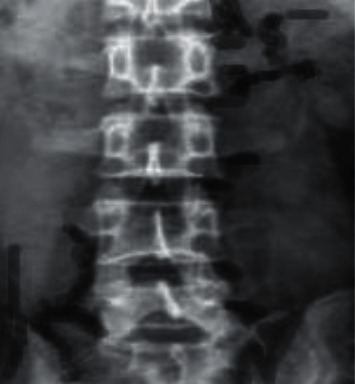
Image under X-ray guidance.
